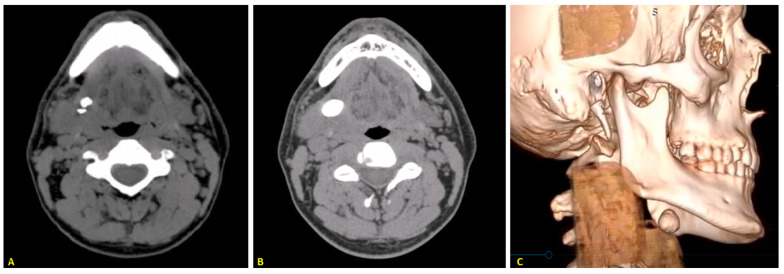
(A) CT scan shows the primary stone in the hilar region and a second, smaller stone located behind it. (B) CT showing the recurrent stone in the same patient after treatment using the slitting and marsupialisation approach. (C) Three-dimensional high-resolution computed tomography reconstruction showing the recurrent stone.
